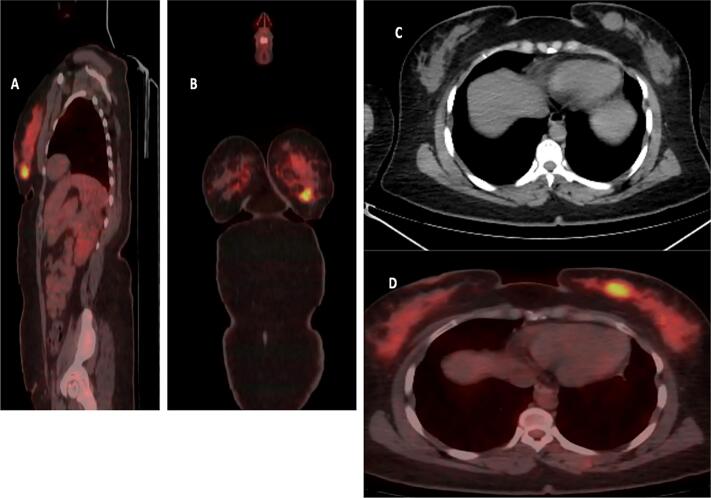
PET-CT scan sagittal (A), coronal (B), and axial (D) images shows a focal intense hypermetabolism in the left breast with standardized uptake value (SUV) max 8 corresponding to a 2.2 × 2 cm nodule in the lower outer quadrant, as seen on computed tomography (CT) scan (C).
